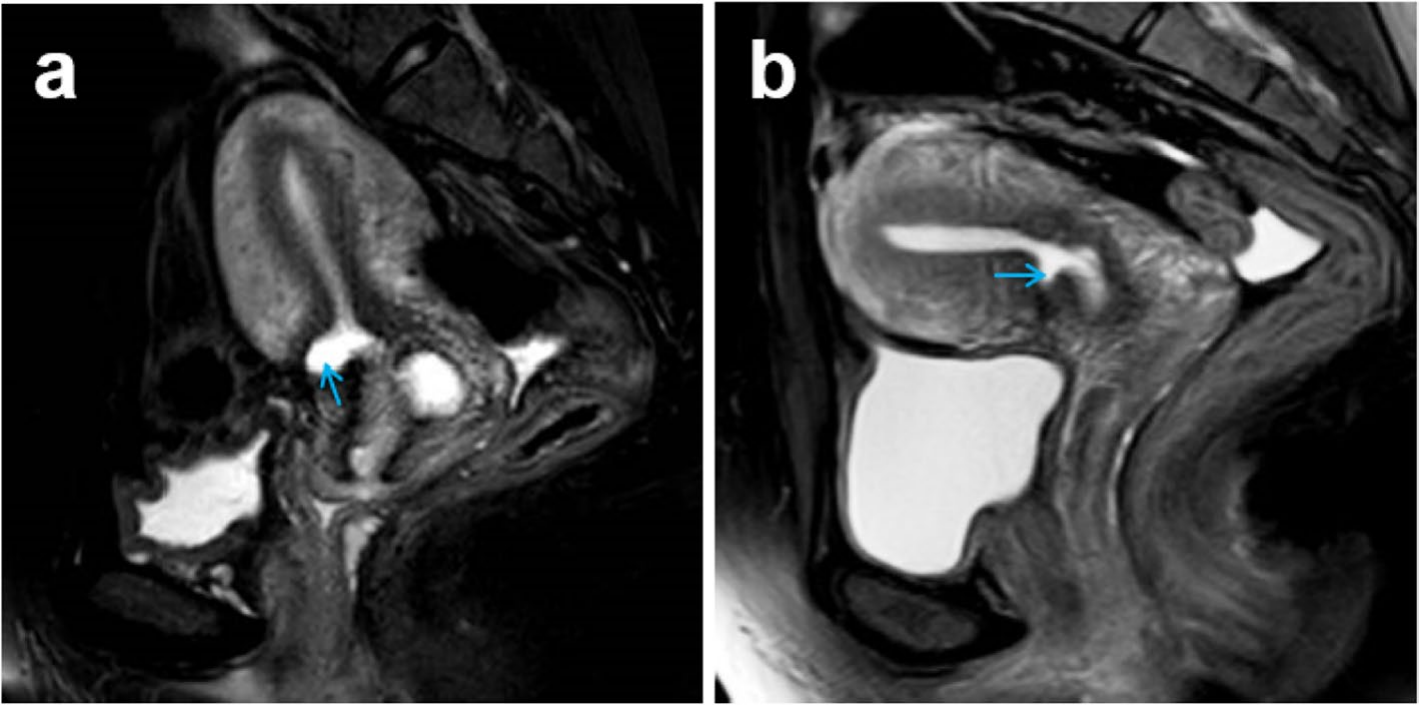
Magnetic resonance imaging result: a before CSD repair; b after CSD repair
